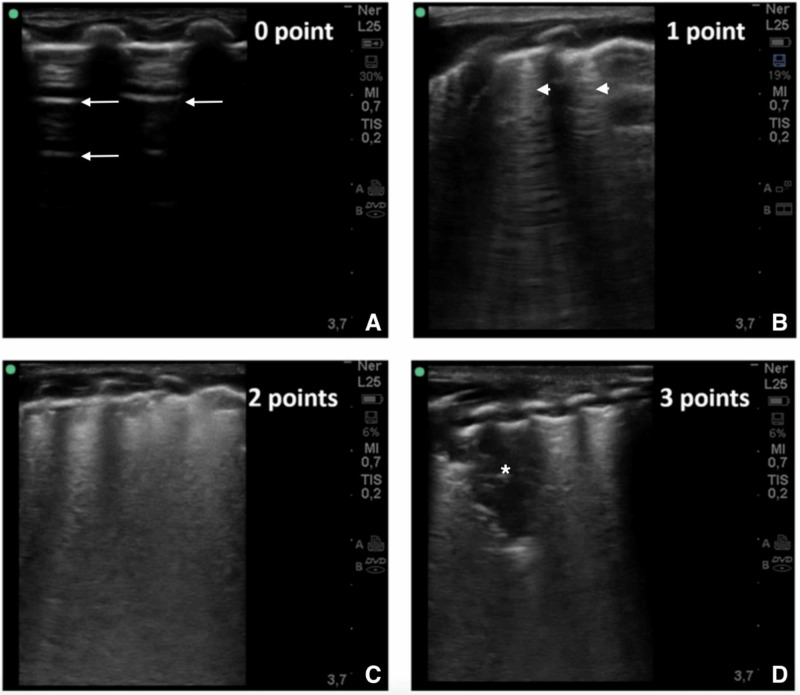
Lung ultrasound scoring and sonographic appearance. A 0 points; normally aerated lung with A line pattern (A-line denoted by arrows) that represents reflections of sonographic waves off the pleura in a non-obstructed air-filled lung. B 1 point; mild interstitial syndrome with non-confluent B-line pattern (B-line denoted by arrow heads) representing transmission of sonographic waves through lung parenchyma with increased density filling the pulmonary interstitial and/or alveolar spaces. C 2 points; severe interstitial syndrome with confluent B-line pattern with or without evidence of consolidation < 1 cm in depth from the pleural line. D 3 points; extensive lung consolidation (Denoted by asterisk) with coalescent B-lines with sub-pleural consolidation ≥ 1 cm in depth from the pleural line extending into the parenchyma.
